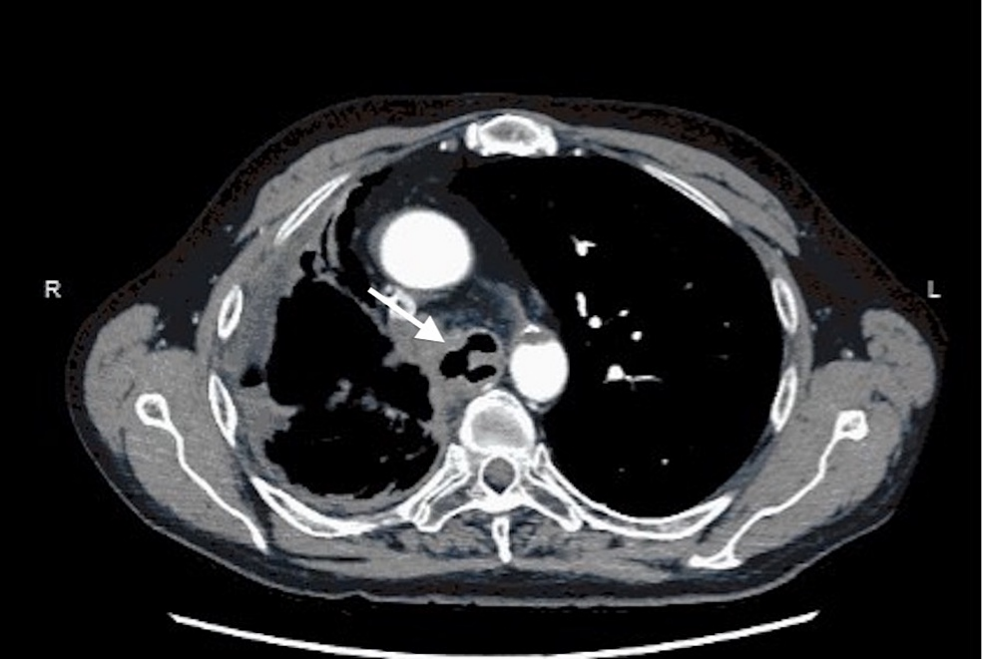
Axial computed tomography image demonstrating the broncho-parenchymal-esophageal fistula (arrow)
